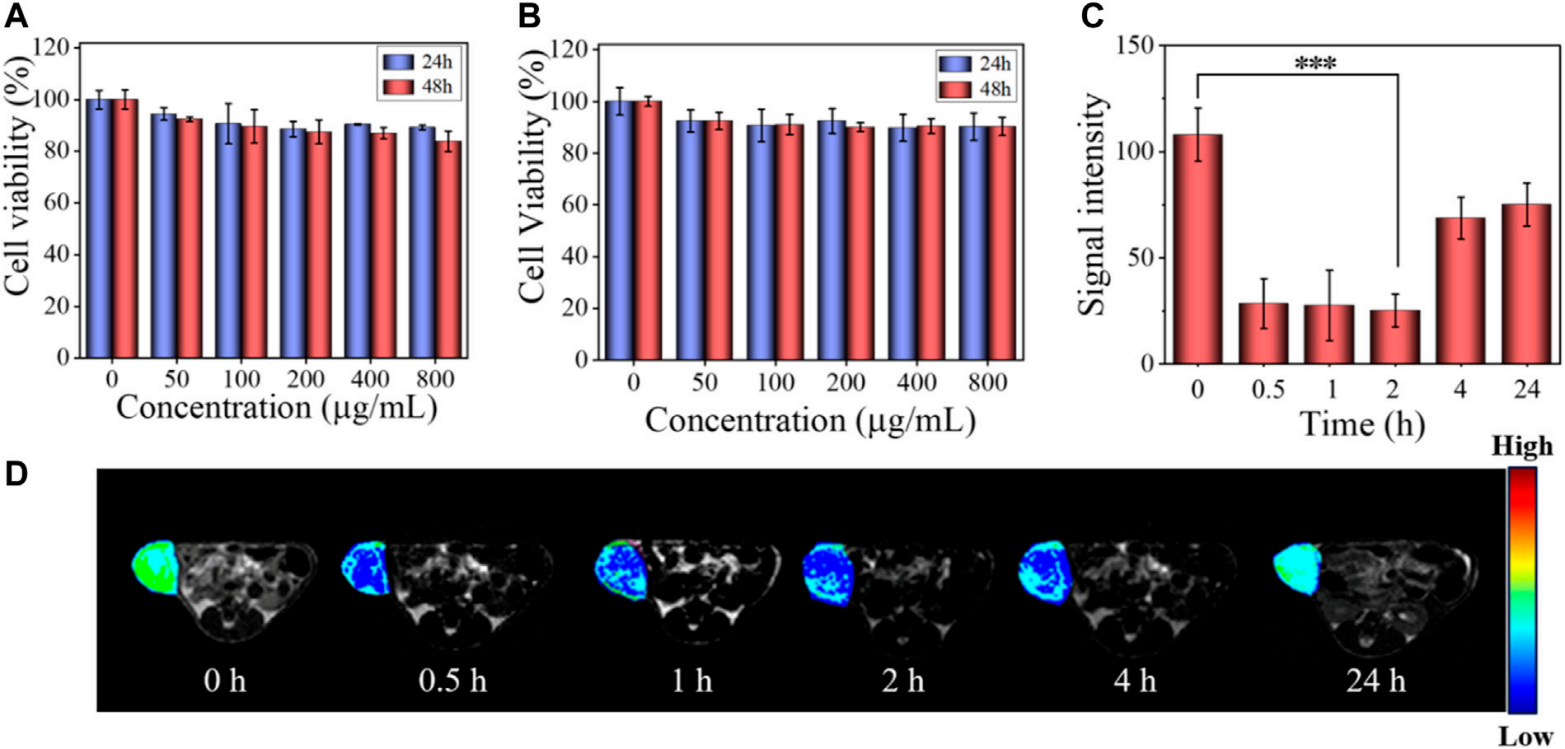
(A) Viability of HeLa cells after incubating with SPNs for 24 and 48 h. The data are given as mean ± SD (*n* = 5). (B) Viability of HeLa cells after incubating with SSPNs for 24 and 48 h (*n* = 5). (C) Signal intensity of *in vivo* MR imaging corresponding to (D) (*n* = 3,p* < 0.001, *p* < 0.01, p* < 0.05). (D)
